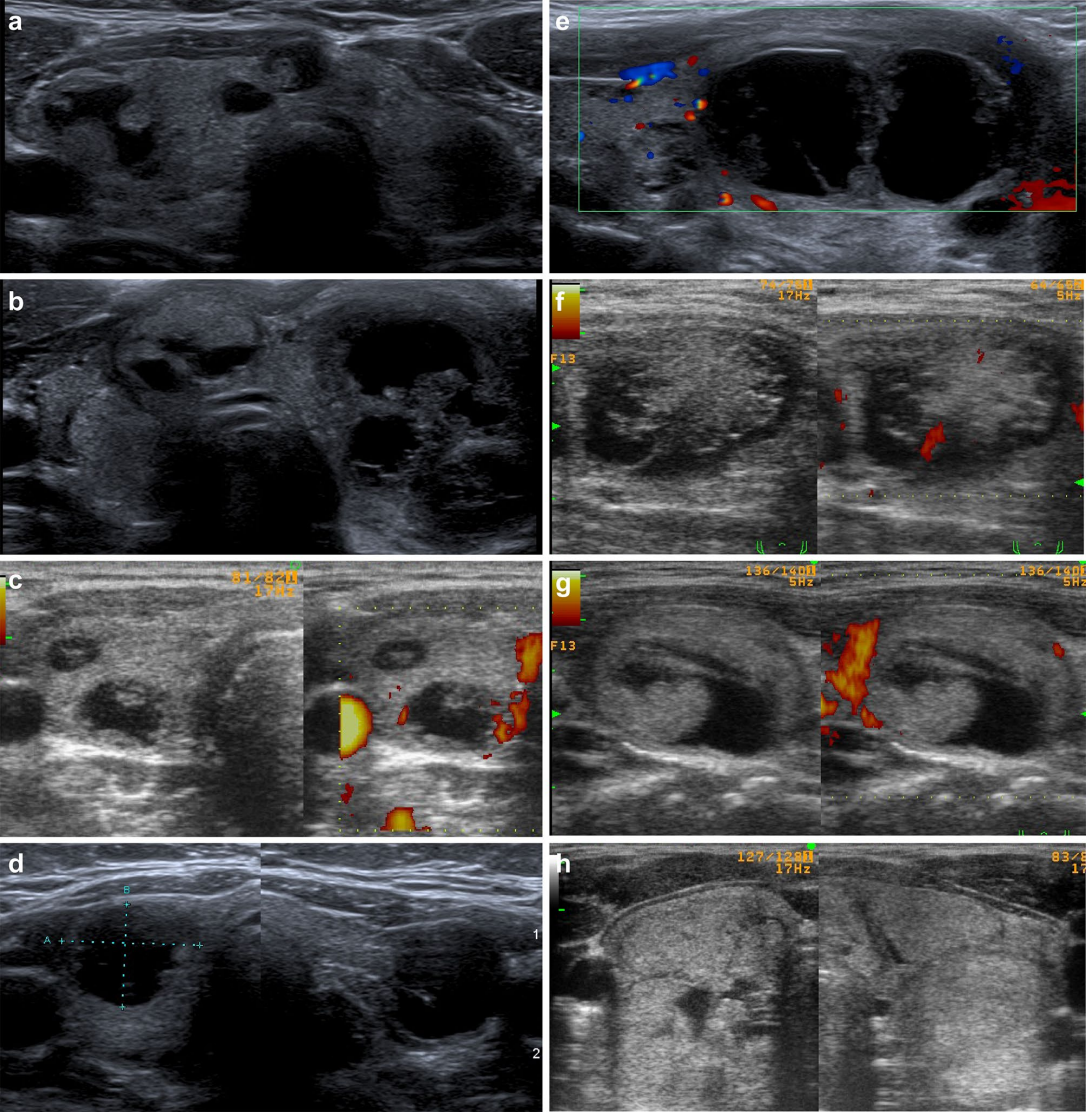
(a-h) Ultrasonographic presentation of MNG in all *DICER1*mut+ pediatric patients. (a–c) A classic image of MNG for DICER1 syndrome—multiple focal lesions within thyroid (“polymorphic mix”) with dominating mixed cystic and solid nodules (type II) and single cystic (type I) or solid (type II) lesions; (d) type I (simple cysts); (e) type Is (septated cyst); (f,g) type II (mixed cystic and solid); (h) type III (solid).
